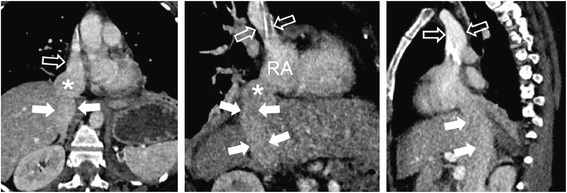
Paracoronal reconstructions of contrast-enhanced CT show normal connection of the superior vena cava (*open arrows*) into the right atrium (*RA*). Connection of the IVC (*arrows*) to the RA, however, is abnormal with angulation and bulbus-shaped configuration (*)
